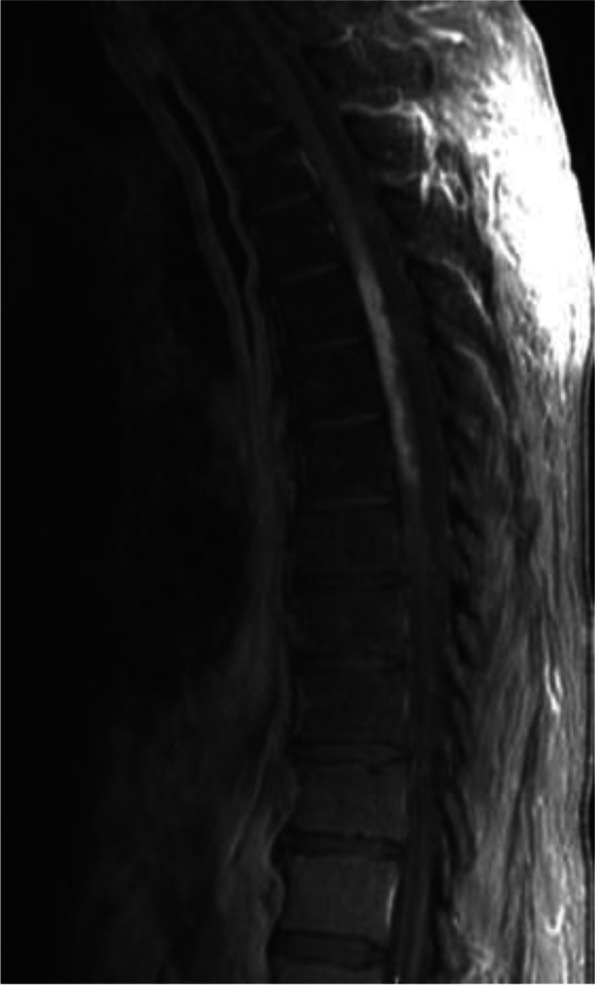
MRI thoracic spine, Sagittal T1 with Gad: showed an enhancing lesion from T3 till T7
